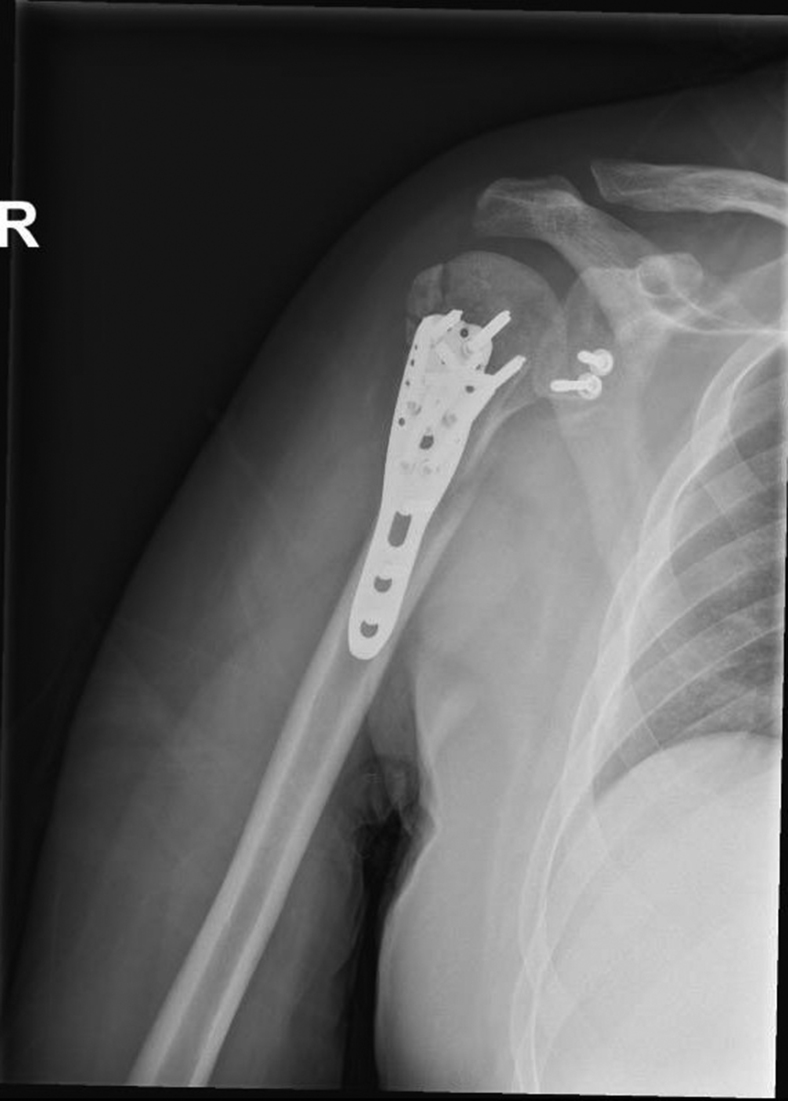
Postoperative lateral radiograph after 6 weeks, showing an adequate position of the humeral head, centrally opposing the glenoid.
